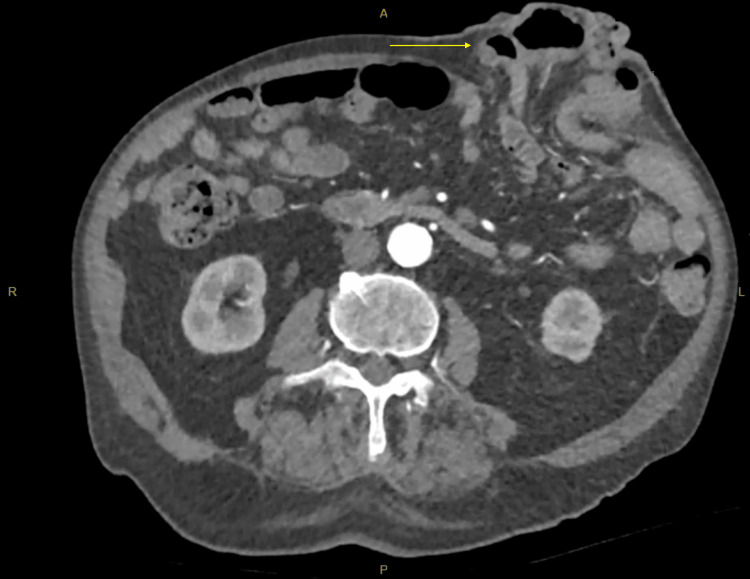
Parastomal hernia following Hartmann’s procedure CT of the abdomen and pelvis image demonstrating a parastomal hernia (arrow) adjacent to the end colostomy following Hartmann’s procedure. The hernia is characterized by a protrusion of abdominal contents through the fascial defect surrounding the stoma site, resulting in a visible bulge of the abdominal wall.
